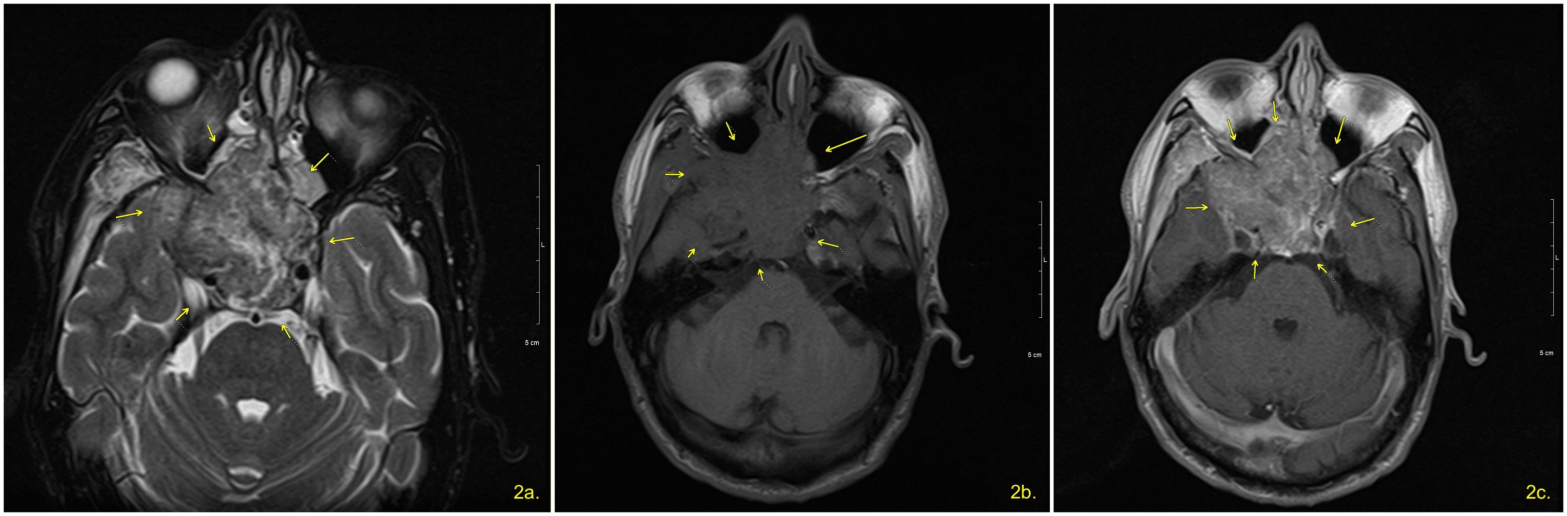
MRI appearance of a heterogeneous, enhancing mass centered within the ethmoid sinus, measuring approximately 6 x 4 cm, involving the mid-posterior ethmoid and sphenoid sinuses with gross destruction of the right greater wing of sphenoid and extension to the right temporal fossa. There is effacement of the right pterygopalatine fat and right Meckel's cave. (2a) Axial T2-weighted MRI demonstrates the mass as heterogeneously hyperintense (yellow arrows); (2b) axial T1-weighted MRI pre-contrast shows the mass as intermediate intensity (yellow arrows); (2c) axial T1-weighted MRI post-contrast demonstrates heterogeneous enhancement of the mass (yellow arrows)
